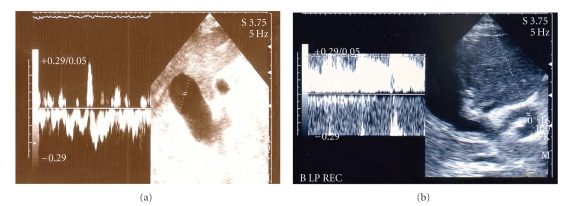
Ultrasonographic images of patient 2 showing (a) two adjacent anechoic circles within the right lob of the liver. The smaller one was dilated right hepatic artery, and the big one was the aneurismatic dilation on the right main branch of portal vein which has turbulent flow within it (b) The dilated and tortuous right hepatic artery ending in the aneurism.
